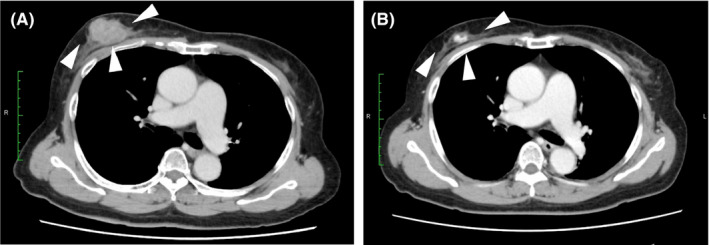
Contrast‐enhanced computed tomography (CT). A, CT before chemotherapy showing the mass in the right breast (arrowhead). B, CT after chemotherapy showing remarkable mass shrinkage (arrowhead)
